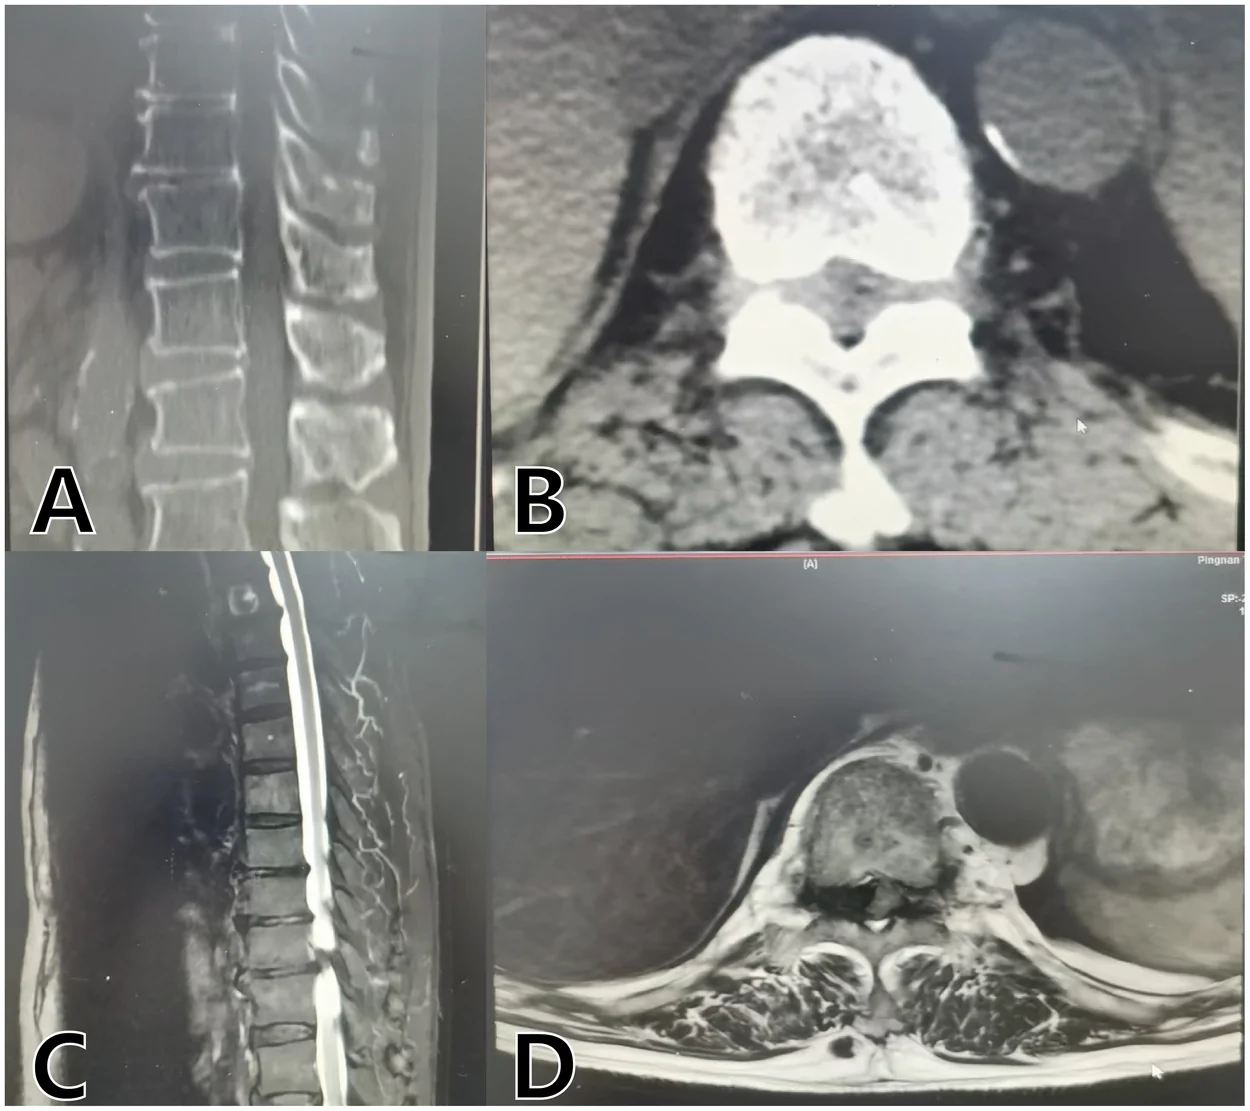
Preoperative images. (A,B) CT image taken at the T10/11 level show thoracic disc herniation. (C,D) MRI scan taken at the T10/11 level show severe spinal cord compression. CT, computed tomography; MRI, magnetic resonance imaging.
